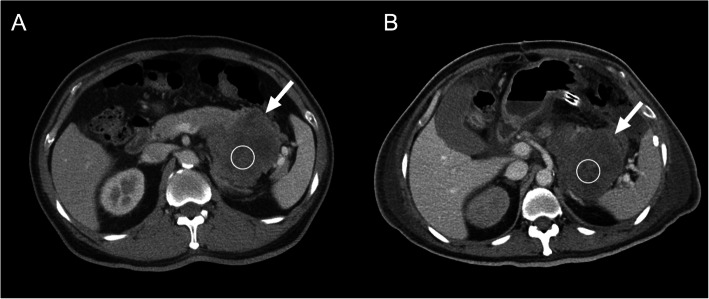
Application of Choi criteria in the gastrointestinal stromal tumor (GIST) after targeted therapy with imatinib. A 49-year-old man was diagnosed with GIST. A Pretreatment CE axial CT scan shows an 88-mm-sized enhancing tumor (arrow) arising from the stomach. The measured CT number on the region of interest (ROI) is 36.1 Hounsfield units (HU). B After targeted therapy with imatinib, posttreatment CE CT scan shows no significantly decreased in tumor size (87 mm in diameter) (arrow) but markedly decreased attenuation (22.6 HU, 37 % reduction), suggesting tumor response according to Choi criteria
